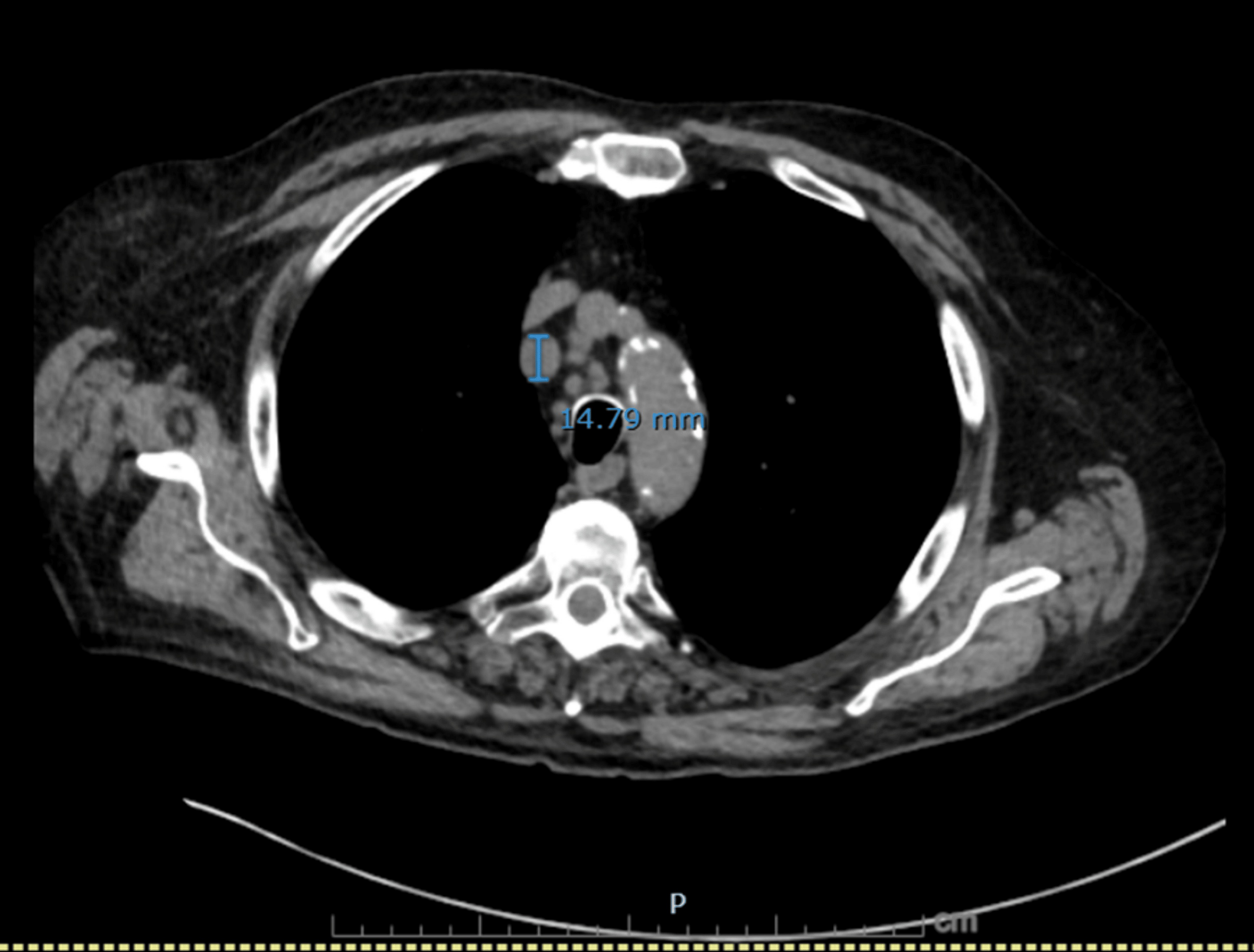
Axial CT chest showing an enlarged right paratracheal lymph node. Axial image demonstrates a right paratracheal lymph node measuring 14.8 mm in short axis, concerning for malignant nodal involvement.
